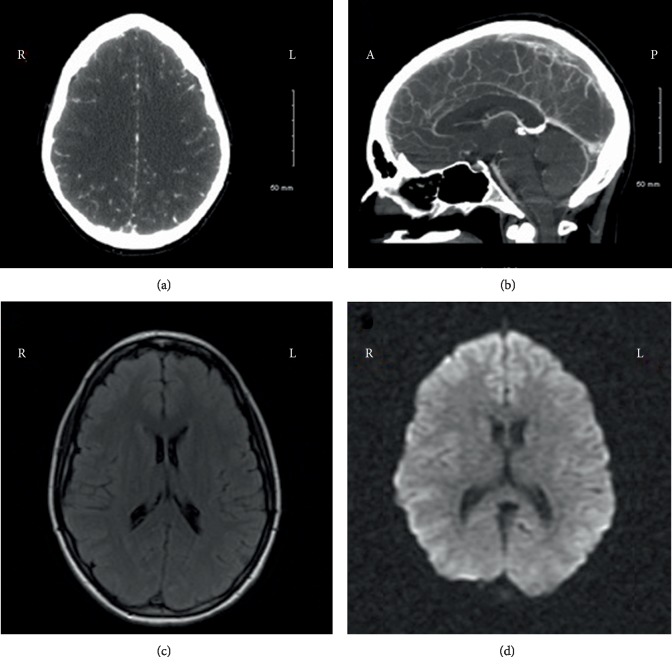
CTV and MRI showing extensive cerebral sinus thrombosis. (a) CT venogram. Axial view. Showing empty delta sign and thrombus in the superior sagittal sinus. (b) CT venogram. Sagittal view. Showing filling defect in the superior sagittal sinus consistent with thrombotic occlusion of superior sagittal sinus. (c) MRI brain T2 Fluid-attenuated Inversion Recovery (FLAIR). Axial view. Showing no parenchymal involvement. (d) MRI brain Diffusion weighted imaging (DWI). Axial view. Showing no acute ischemia or cytotoxic injury.
